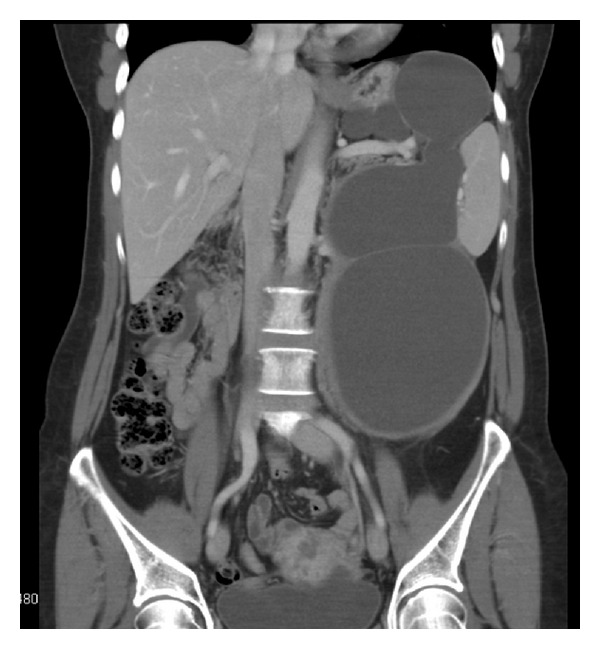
CT scan of the abdomen (coronal view) showing a large cystic mass extending from the diaphragm to the left iliac region.
